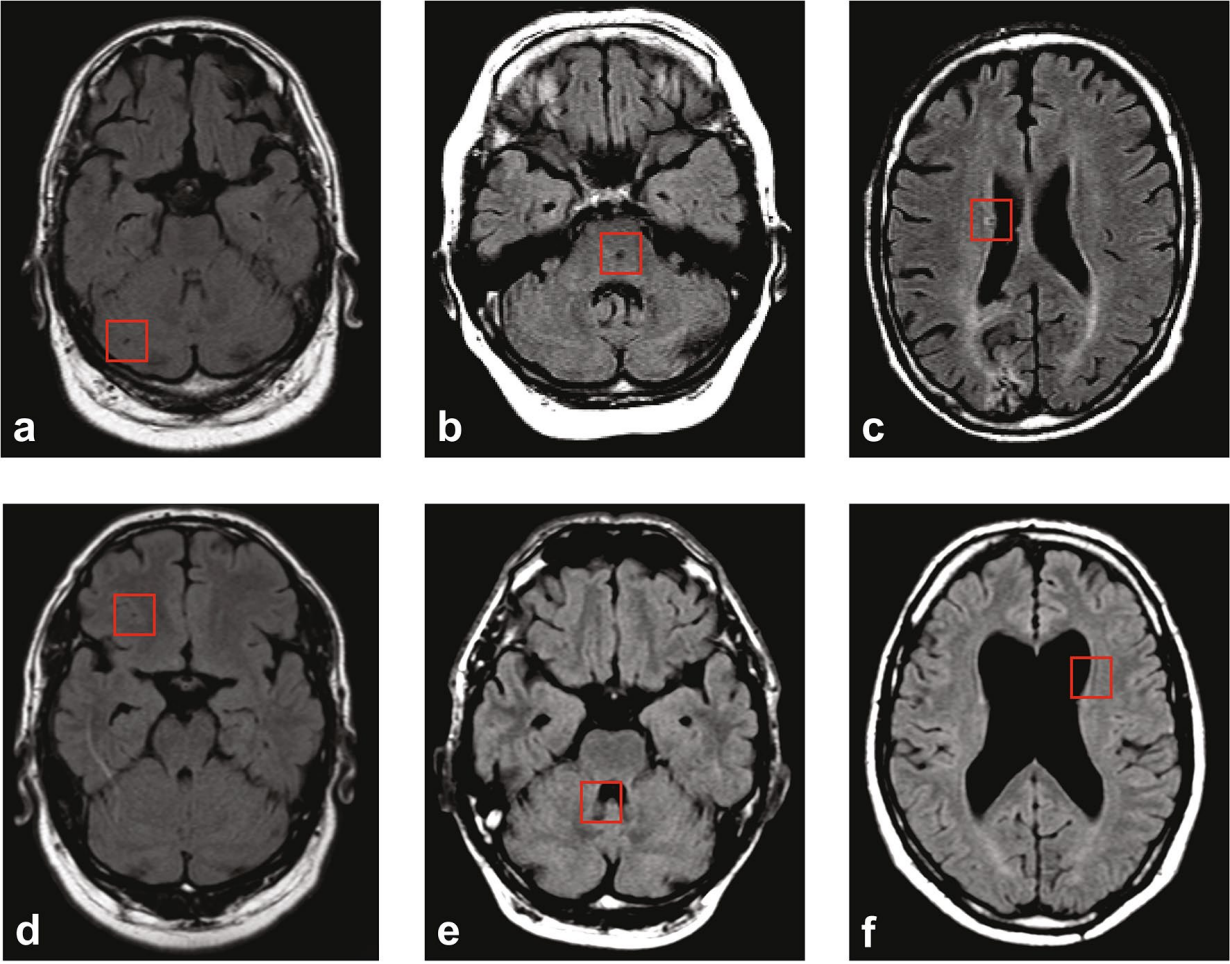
Comparison between missed brain infarcts and nearest neighbor locations in training images. (a–c) Missed brain infarcts indicated by the red square in the cerebellum, brainstem and cerebrum, respectively, on transversal T2-FLAIR images. (d–f) the corresponding nearest neighbor locations in training data matching (a–c) respectively. The square in (d) contains a part of a normal sulcus that has a similar appearance as the missed brain infarct in the cerebellum in (b).
